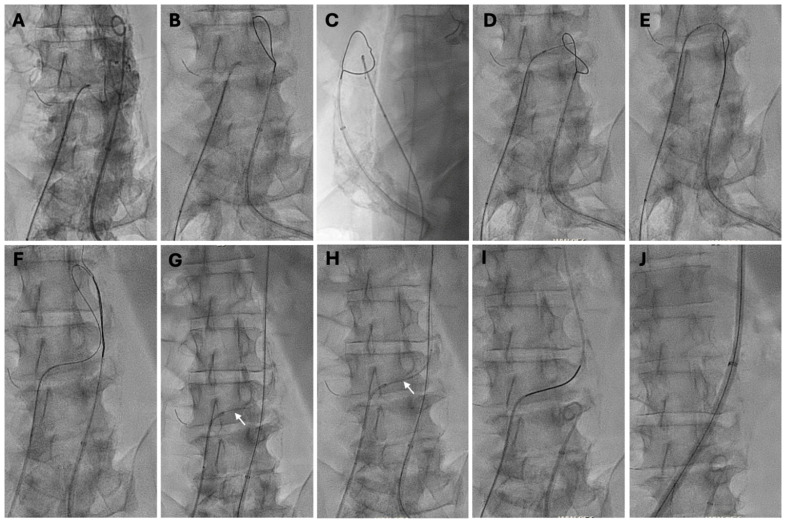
Transcaval Access (A) Traversal assembly at the site predetermined by computed tomography angle, and aortogram via the pigtail catheter. (B) Snare is positioned across the traversal system. (C) Position of the snare across the traversal system is confirmed in the orthogonal en face projection. (D) 0.014-inch guidewire is traversed across the vena cava and aorta into the snare with electrosurgery. (E) The aortic guidewire is ensnared while feeding forward to avoid outward traction on the aortic catheter. (F) The aortic guide is advanced, leading with a looped guidewire. (G) 0.014-inch microcatheter (arrow) is advanced over the 0.014-inch guidewire. (H) A 0.014-inch microcatheter (arrow) creates a 0.035-inch caval-aortic rail to deliver a 0.035-inch microcatheter. (I) A stiff 0.035-inch guidewire is delivered up to the aortic arch through the 0.035-inch microcatheter. (J) The transcatheter aortic valve replacement introducer sheath advances into the aorta.
